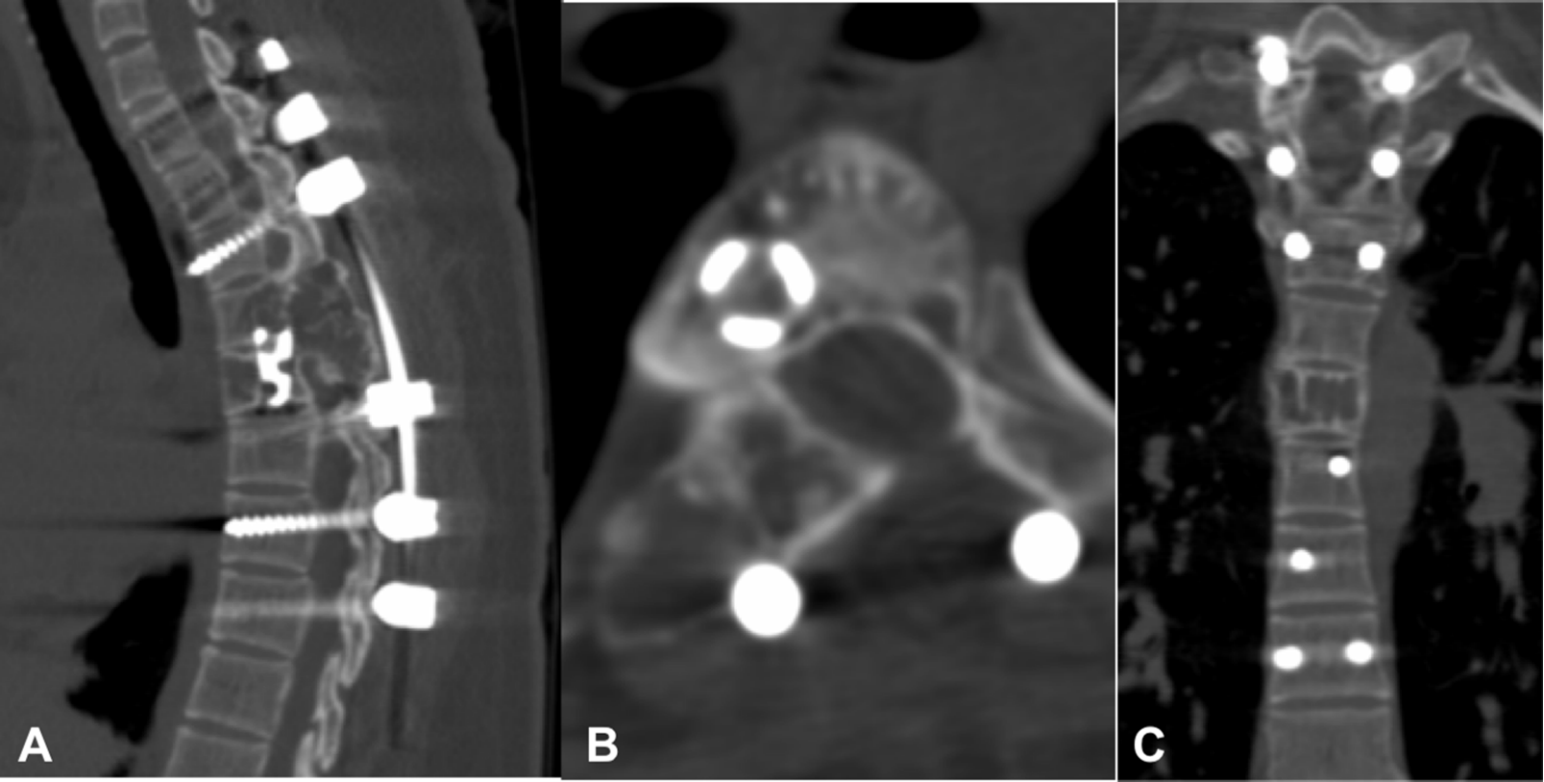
2-year follow-up CT scan. Ossification of the lesion with remodelling of the spinal canal and incorporation of Harm’s cage seen on Sagittal (A), axial (B) and Coronal (C) images.
